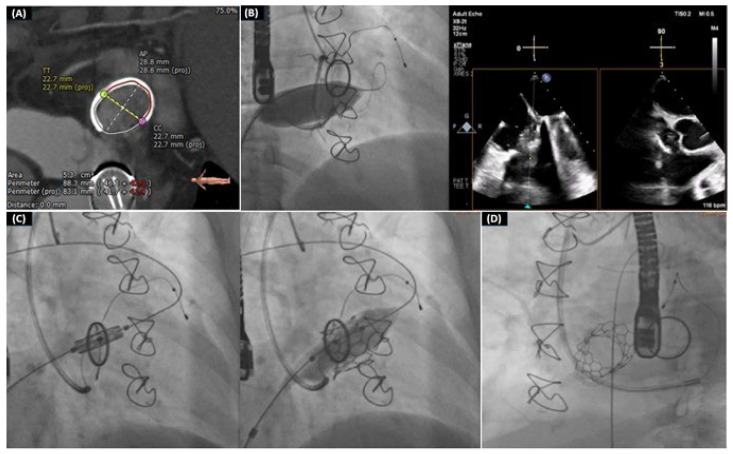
This is the case of a 53-year-old female patient with progressive dyspnea (NYHA III), past medical history of breast cancer (mastectomy, chemotherapy, and radiation therapy), surgical MV management with mechanical prosthesis (St Jude 23 mm), and tricuspid valve repair with annuloplasty ring (Edwards physio ring 28 mm). This figure presents the valve-in-ring’s procedure steps: (A) pre-procedural screening with analysis of the cardiac computed tomography, (B) balloon testing for right coronary artery occlusion, (C) transcatheter heart valve implantation, (D) final result.
